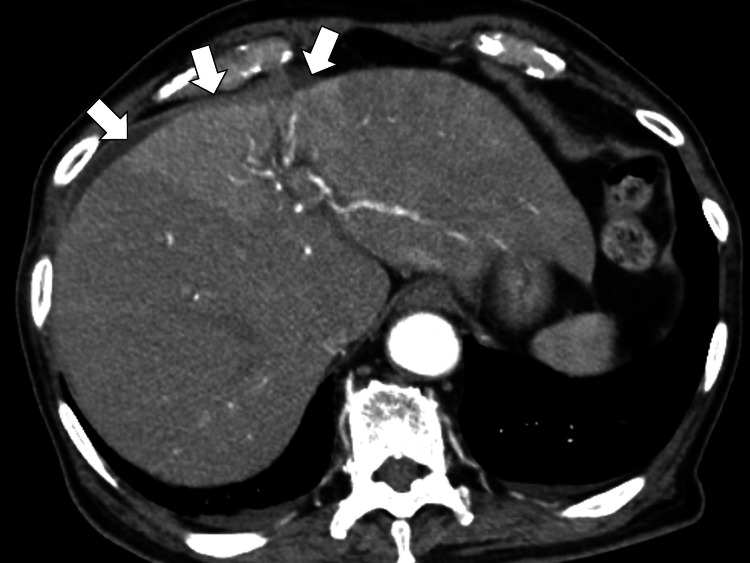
Contrast-enhanced computed tomography of the abdomen revealing a partial enhancement of liver and intrahepatic bile ducts (white arrows).
